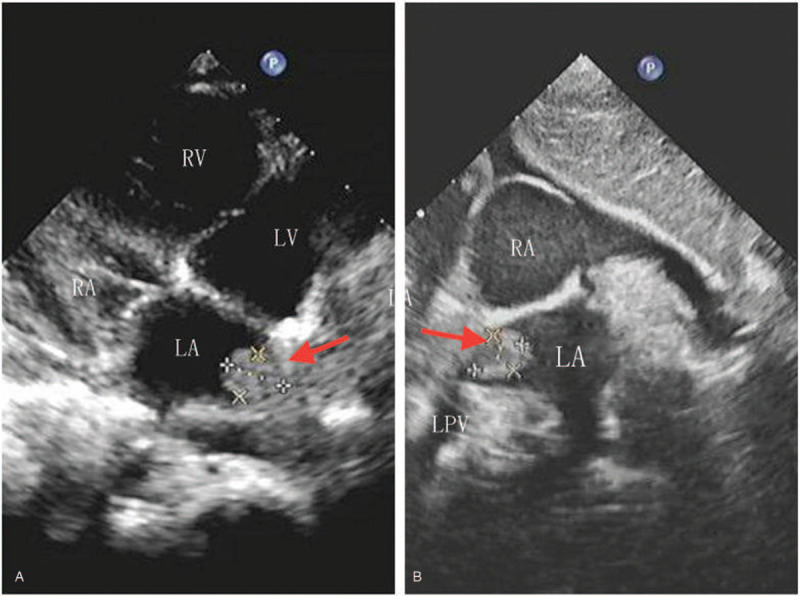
Echocardiography. The echocardiography revealed the thrombus attached to the lateral wall of the left atrium extending into the right lower pulmonary vein. LA = left atrium, LV = left ventricular, RA = right atrium, RLPV = right lower pulmonary vein, RV = right ventricular.
